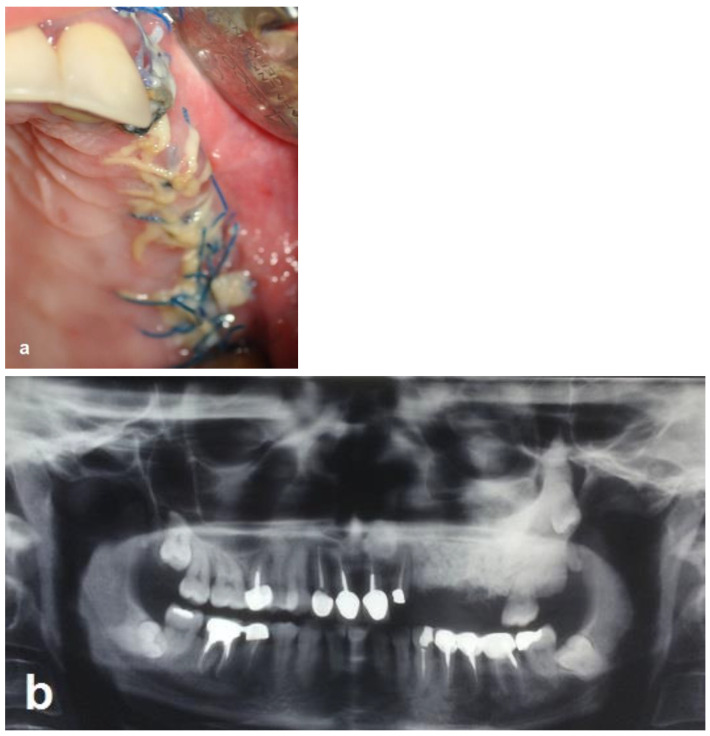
Follow-up 4 weeks after the operation. (a) Clinical view shows the maintenance of the primary closure without wound dehiscence. (b) Radiographic view shows the augmented area and the difference in opacity.
